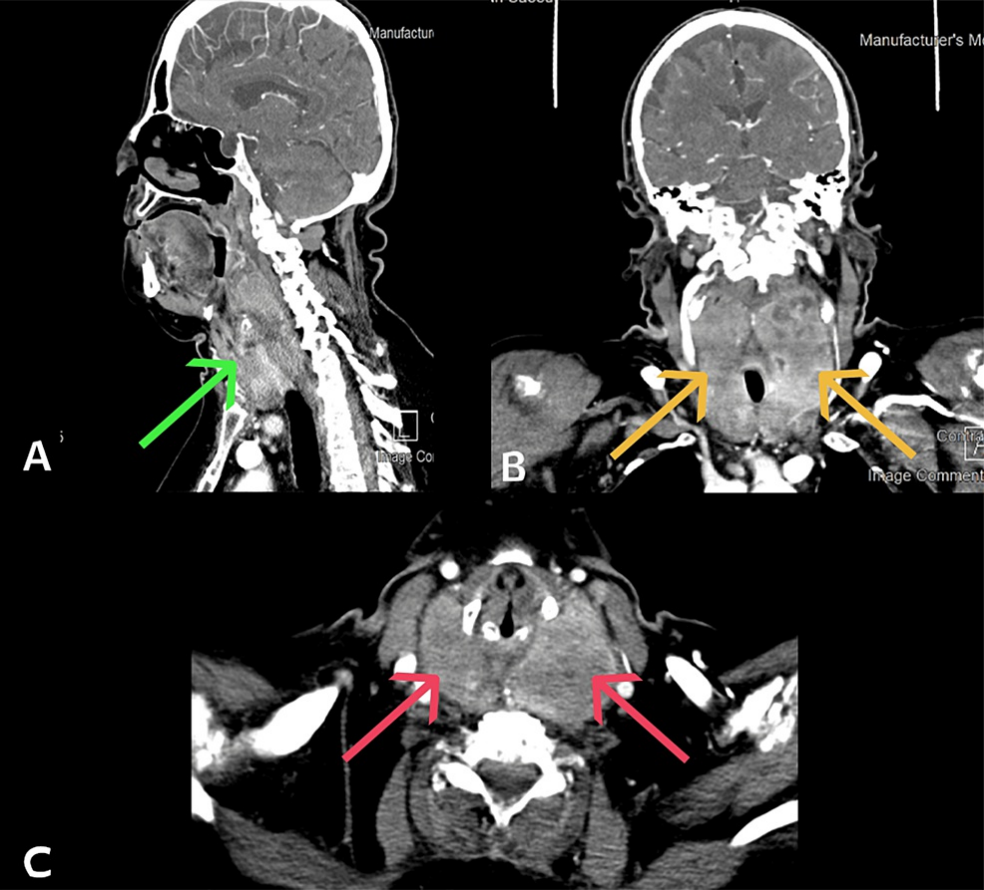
(A) A sagittal CT of the neck shows a thyroid mass; (B) an axial CT of the neck shows a bilateral thyroid mass; and (C) a coronal CT of the neck shows a bilateral thyroid mass. A: The green arrow shows a thyroid mass with no retrosternal extension; B: The orange arrow shows a bilateral thyroid mass with a significant mass effect on the trachea; and C: The red arrow shows a bilateral thyroid mass with a significant mass effect on the trachea.
